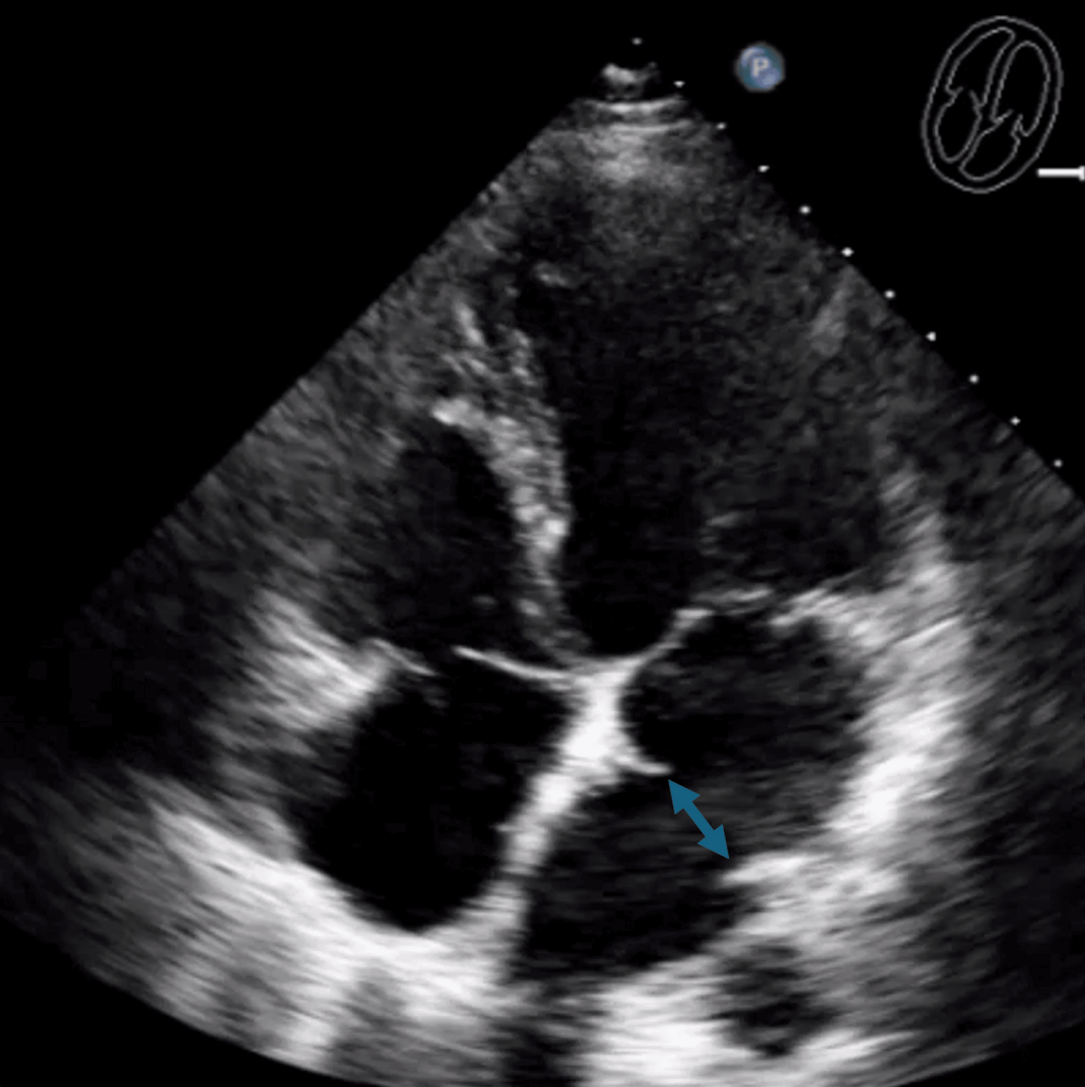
TTE showing an abnormal septum within the LA (double-headed arrow) LA, left atrium; TTE, transthoracic echocardiogram
